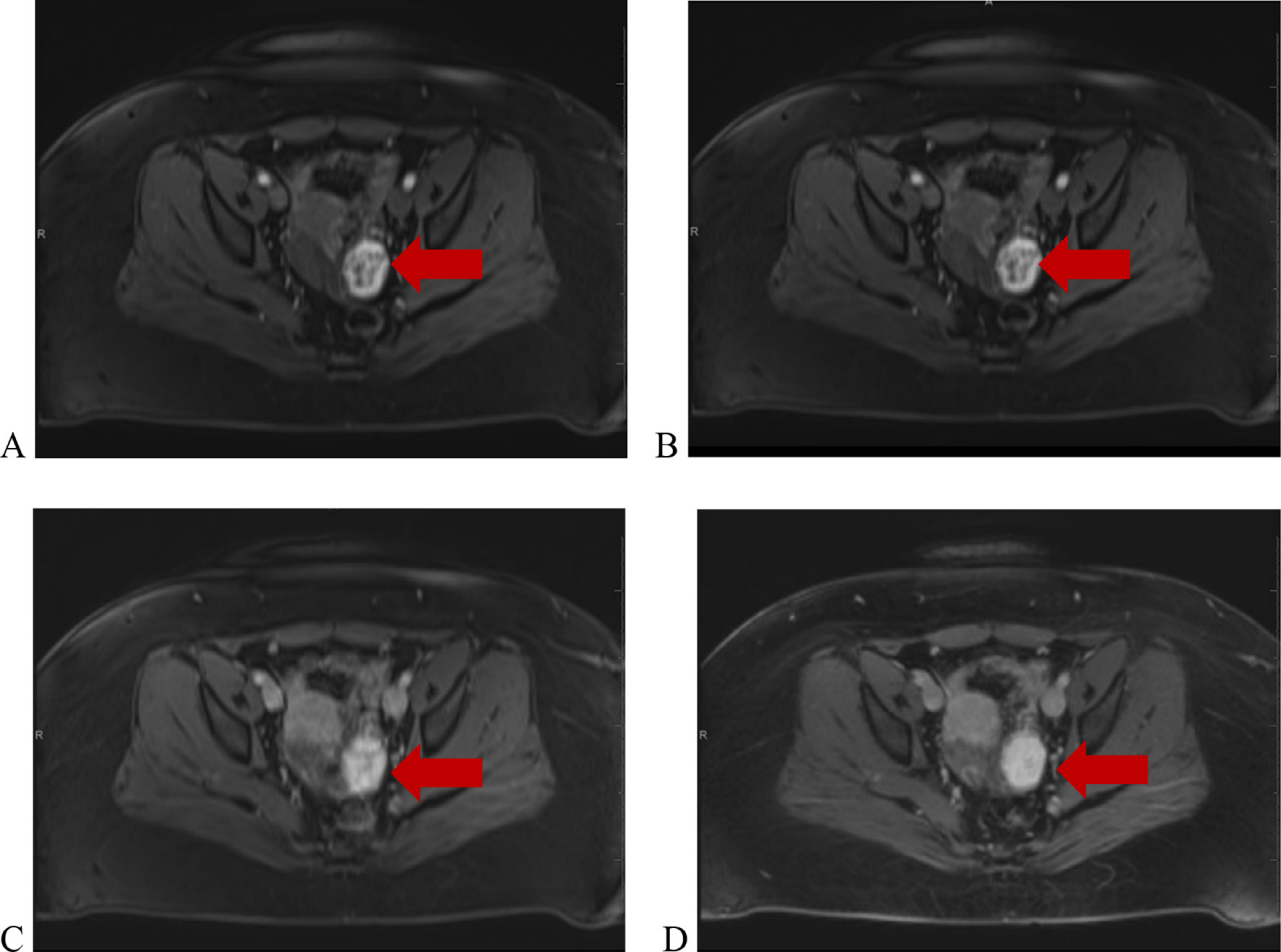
(A-D) MRI of the pelvis. Axial post-contrast T1-weighted images demonstrate avid enhancement with progressive centripetal “filling-in” at 30, 60, 90, and 120 seconds.
